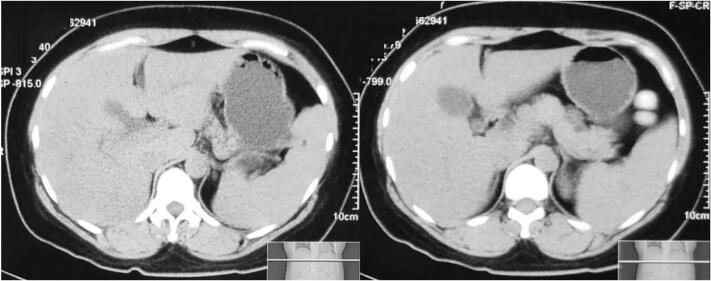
Pre-operative CT-scan showed cholelithiasis and cholecystitis as well in the left lobe intrahepatic biliary duct dilatation, and cystic duct dilatation.
